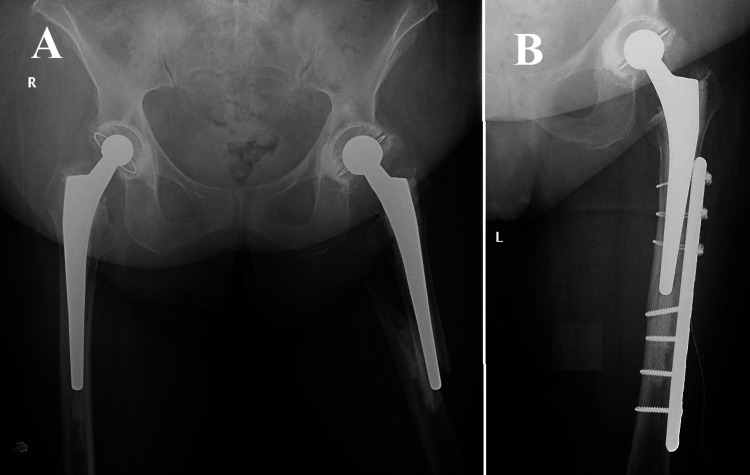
Vancouver B1, Case one One of the instances that exemplify the intricacy of Vancouver B1 periprosthetic femoral fractures involved a 76-year-old individual who had previously received cemented total hip arthroplasty eight years ago. (A) Surgery was required as per the patient's age, cemented stem stability, and fracture characteristics. A specialized plate with distal screws and proximal cerclage wires stabilizes the fracture after an open reduction procedure; (B) Post-surgery radiographs confirm reduction and fixation. These results match the recommended fracture treatment for older patients with stable, cemented prostheses.
